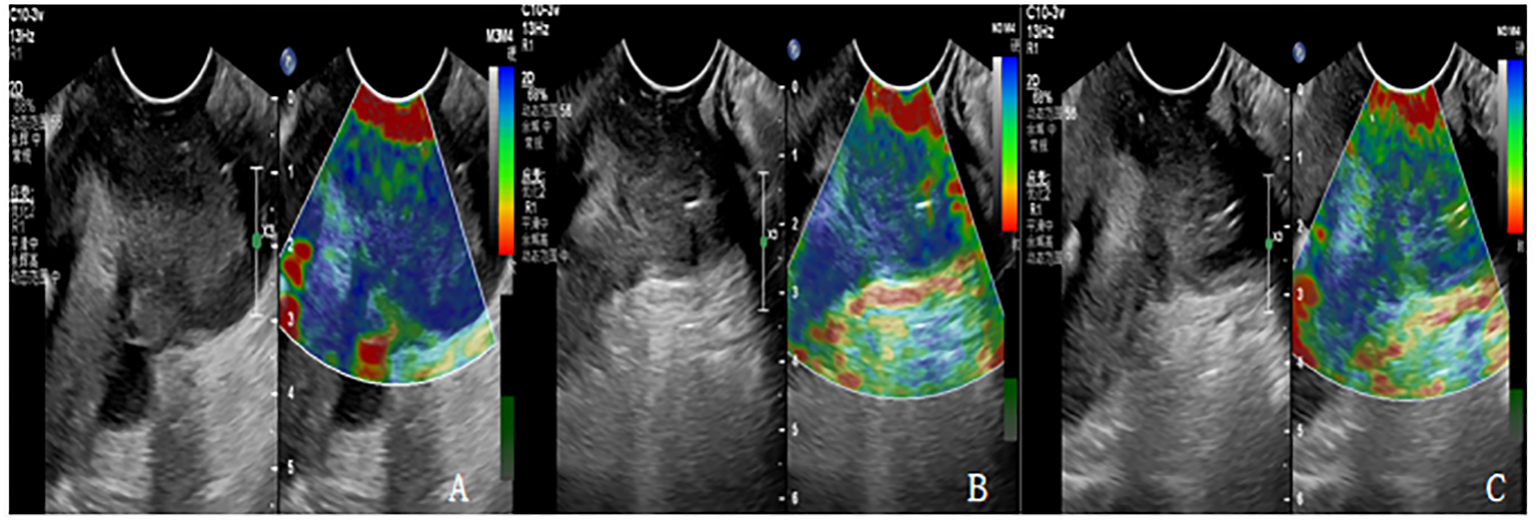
(A-C) are the Ultrasonic elastography of patients with cervical cancer at each time point of CCRT. (A) The lesion had hard elasticity and was basically blue, with an elasticity score of 5 and an SR value of 4.3. (B) The lesion had soft elasticity, blue in the center and a little green around it. The elasticity score was 4 points and SR value was 3.1. (C) The lesion had soft elasticity and appeared blue and green, with an elasticity score of 3 and an SR value of 2.4.
